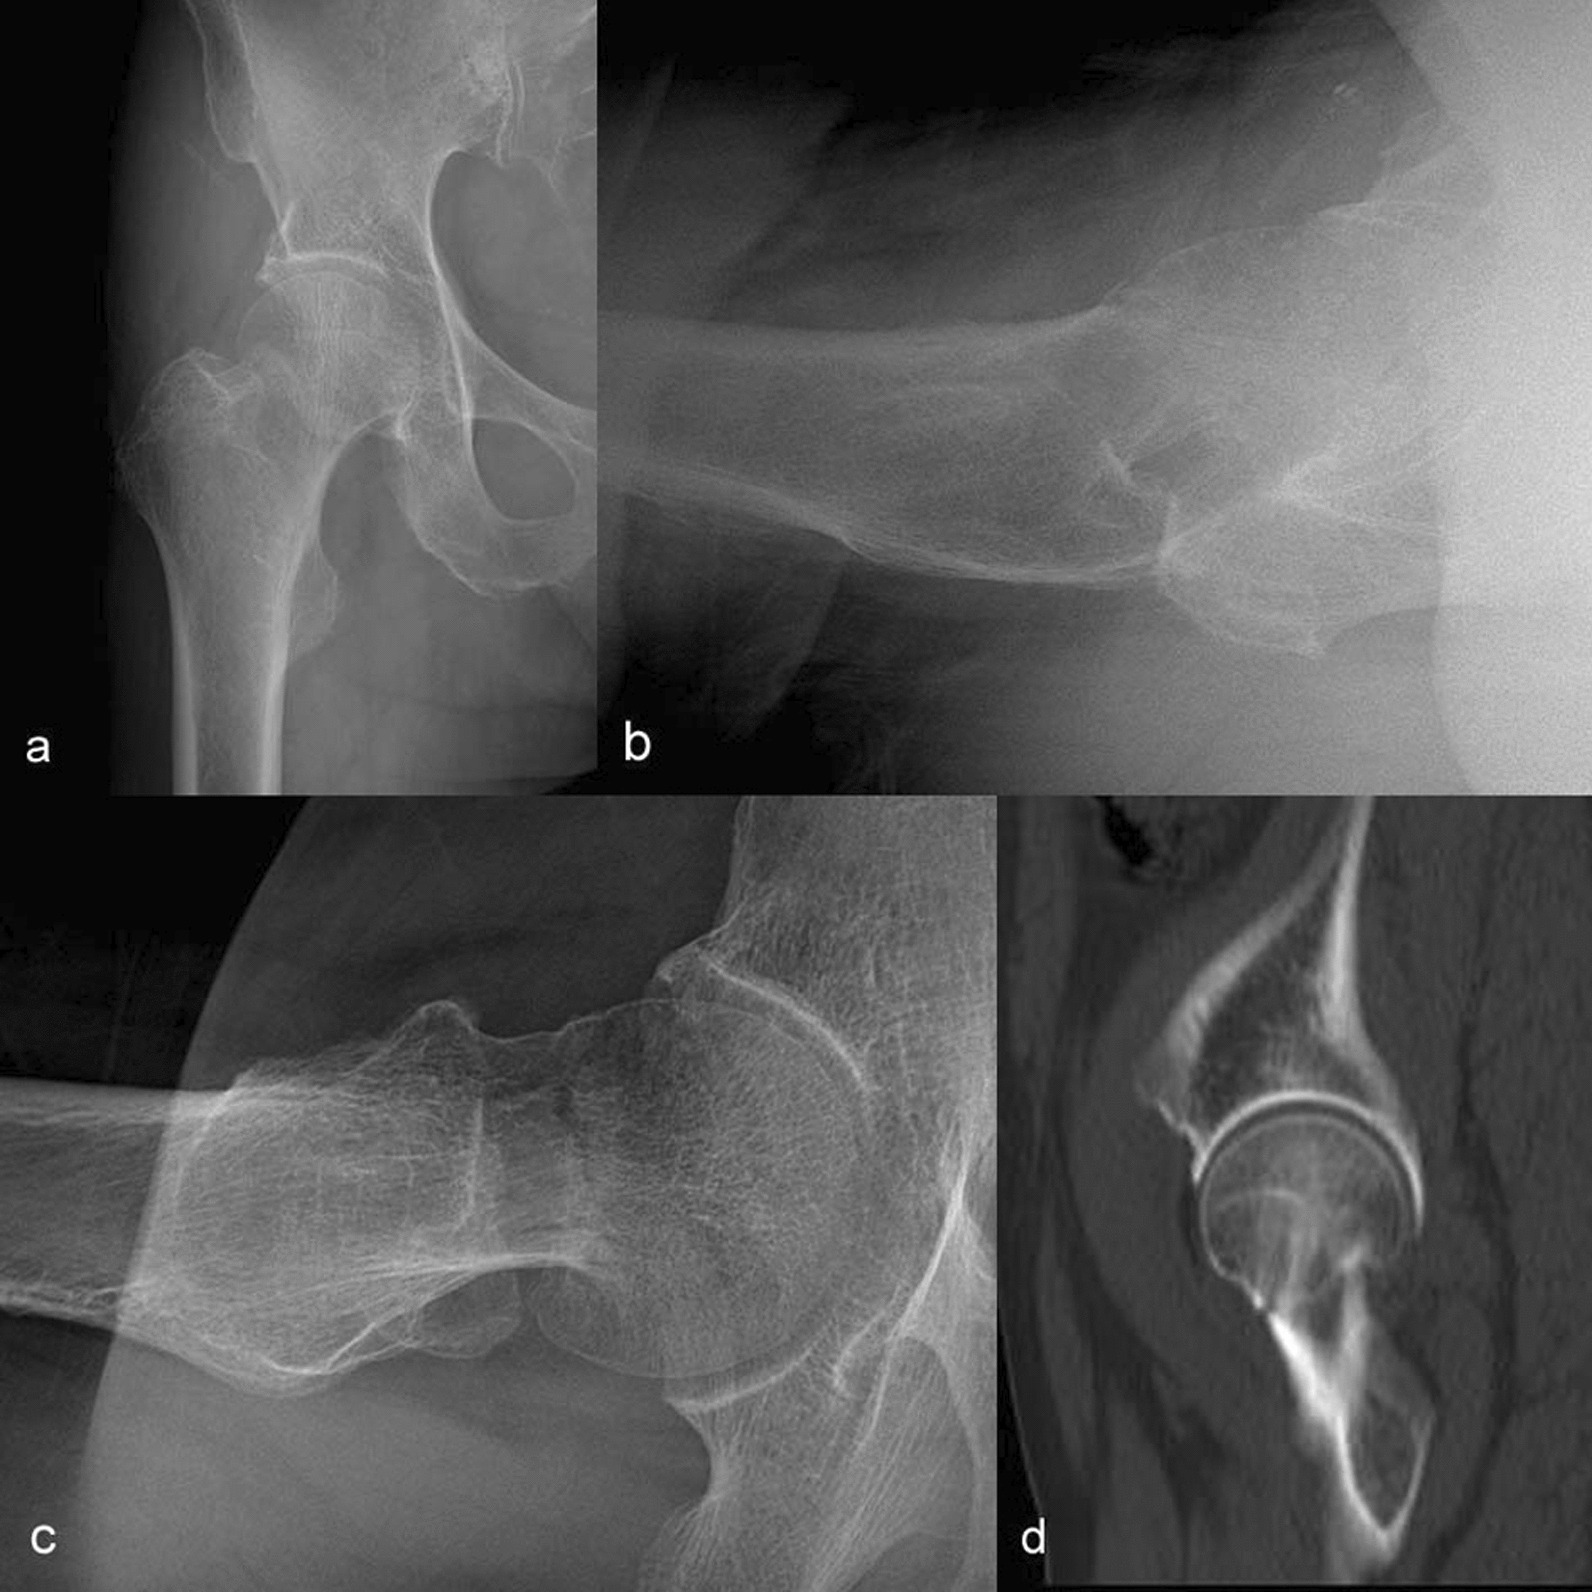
Case 1, a 73-year-old female patient, was admitted to hospital after injury. a, b show the fracture line of femoral neck faintly anteroposterior projection (AP) and cross-table lateral view of hip. c Fracture line of femoral neck clearly in the modified lateral view. d CT imaging, it confirms femoral neck fracture
